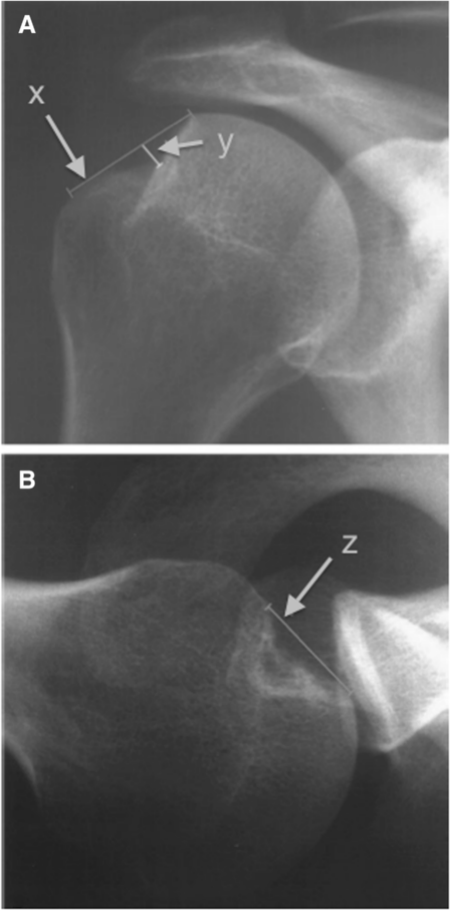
Hill-Sachs Quotient. a) True AP x-ray of the humerus with the shoulder in 60° internal rotation to measure the width (x) and depth (y) of the lesion. b) Bernageau profile view to measure the length (z) of the lesion. The Hill-Sachs Quotient is calculated by multiplying x, y and z. Grade: I <1.5 cm^2^; II 1.5-2.5 cm^2^; III > 2.5 cm^2^ (Adapted from Kralinger *et al.* [39])
